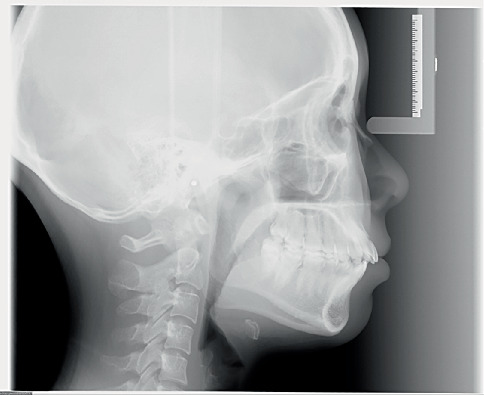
Pretreatment cephalometric x-ray image.
